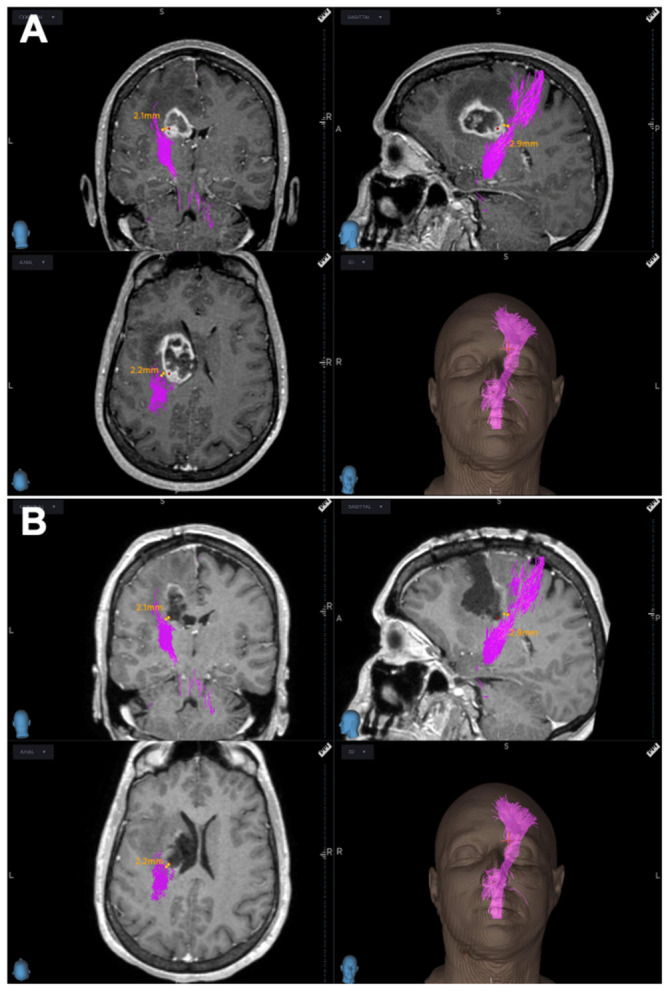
The same distance (in millimeters, mm) between the posterior margin of the tumor and CST (pink) was detected both (A) intraoperative and (B) on post-operative MRI, showing the lack of intraoperative brain-shift effect on CTS reconstructions.
